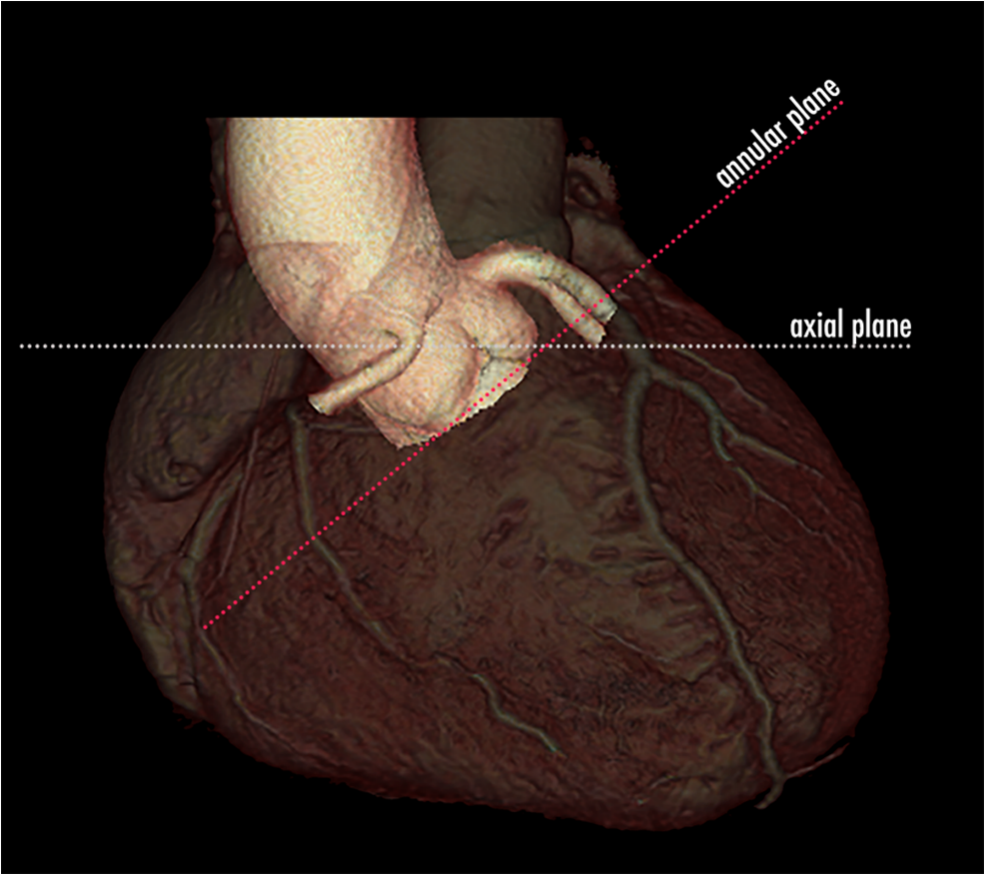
3D volume rendering CT image of the heart containing the aortic root. The aortic root has a double-oblique orientation within the heart. Therefore, standard orthogonal imaging planes, like the axial plane indicated with the dotted white line, are not suitable to correctly visualise the aortic root and containing structures. For this reason, intrinsic 3D imaging modalities like CT are necessary to correctly assess the aortic root and annulus and obtain accurate measurements
